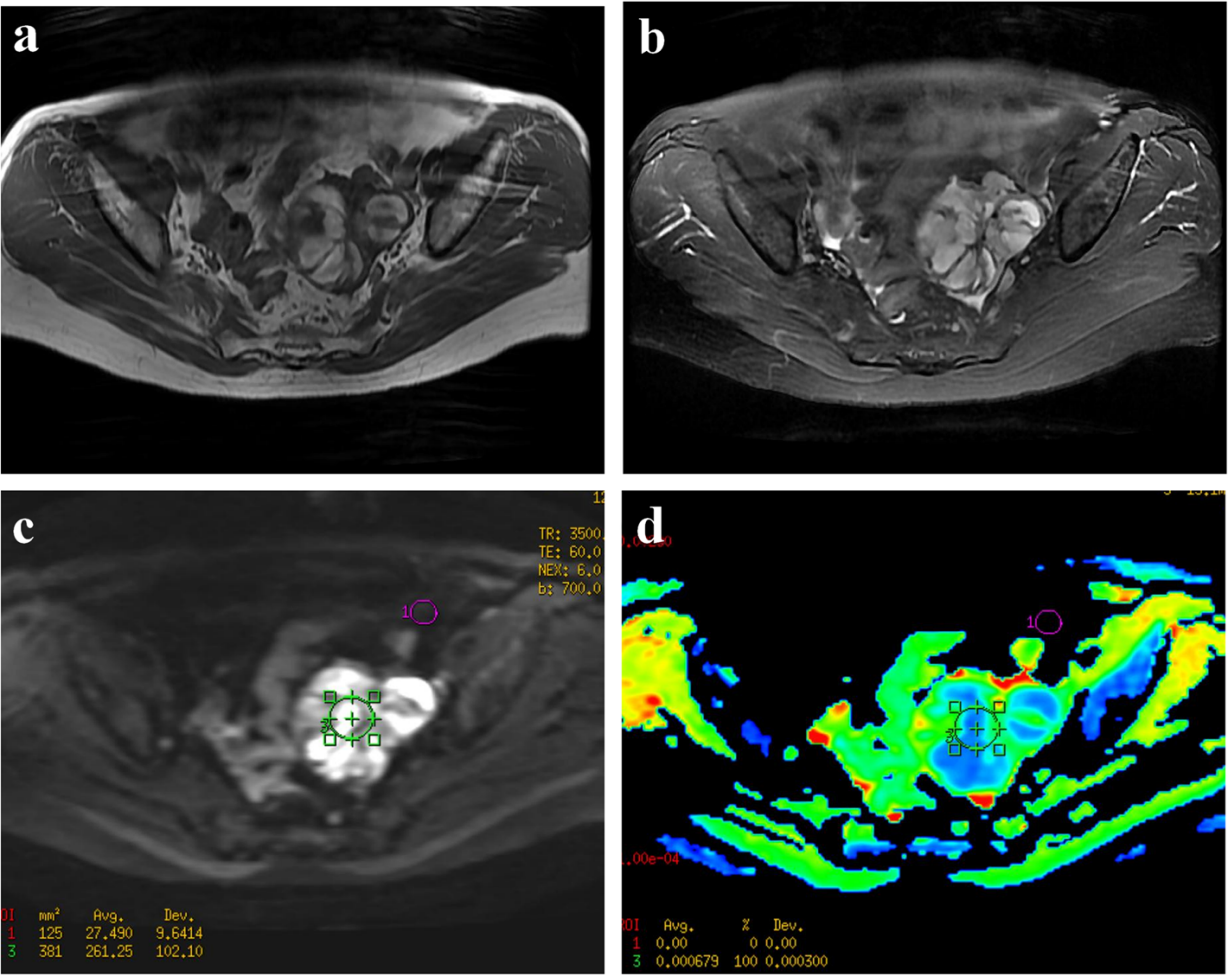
A 60-year-old female patient with Granular cell tumor. (a) Axial T1WI reveals a well-demarcated solid tumor with hyperintensity in the left adnexal region. (b) On fat-suppressed T2WI, the mass shows mixed hyperintensity. (c) On DWI-MRI (*b* = 700 s/mm^2^), the lesion appears as the inhomogeneous hyperintensity. (d) ADC map demonstrates marked hypointensity of the solid components. The ADC value at the corresponding site is 0.68x10^-3^.
